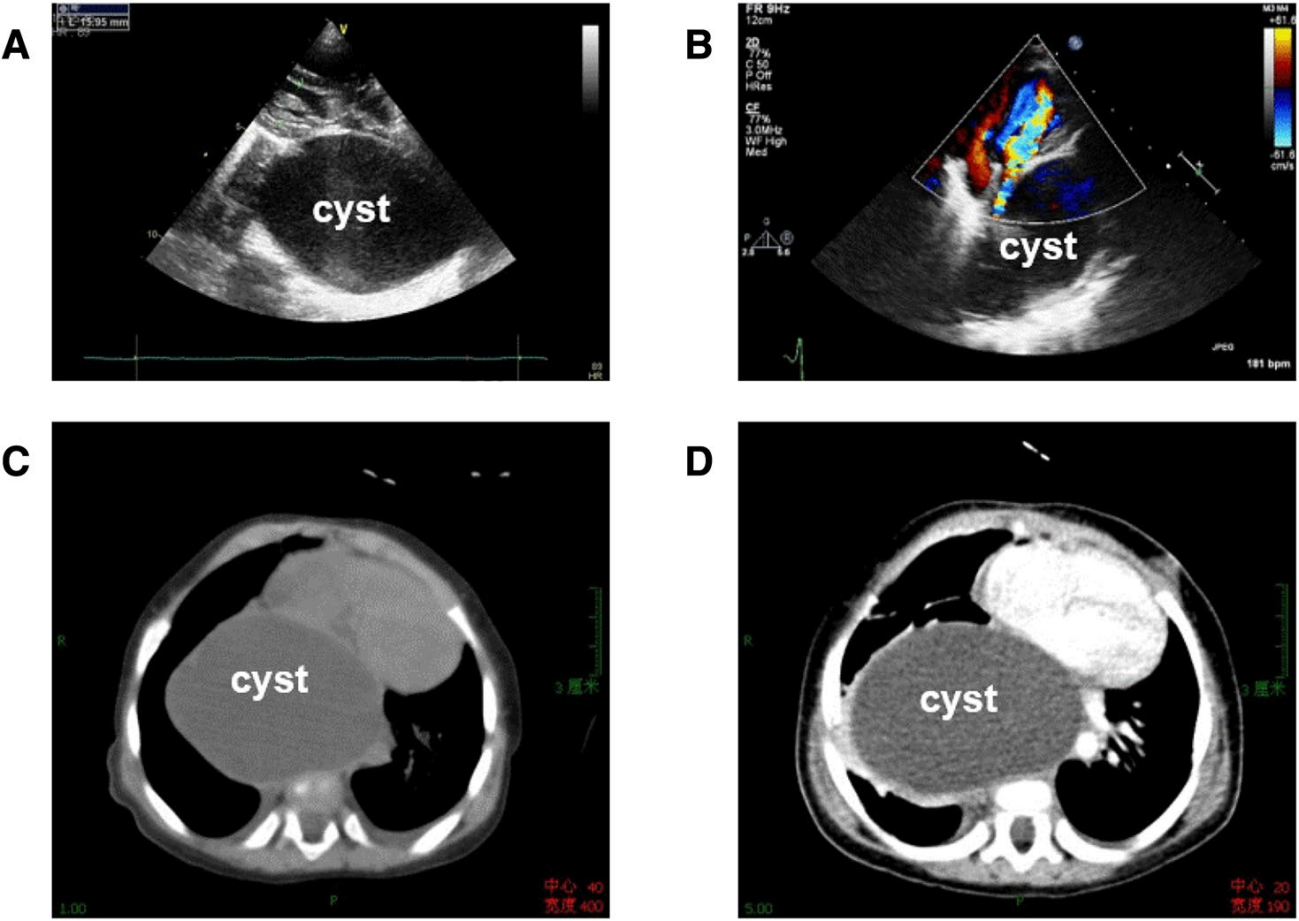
A 6-months-old female infant with a huge enteric cyst, repeatedly coughing for more than 10 days. a Parasternal long-axis view of the left ventricle showed a huge cystic structure behind the left atrium, compressing the atrium obviously; b the apex four-chamber view also indicated the compressive deformation of left atrium; c, d non-contrast CT (c) and contrast-enhanced CT (d) also demonstrated that a large cyst compressed the atrium and it had no blood perfusion
